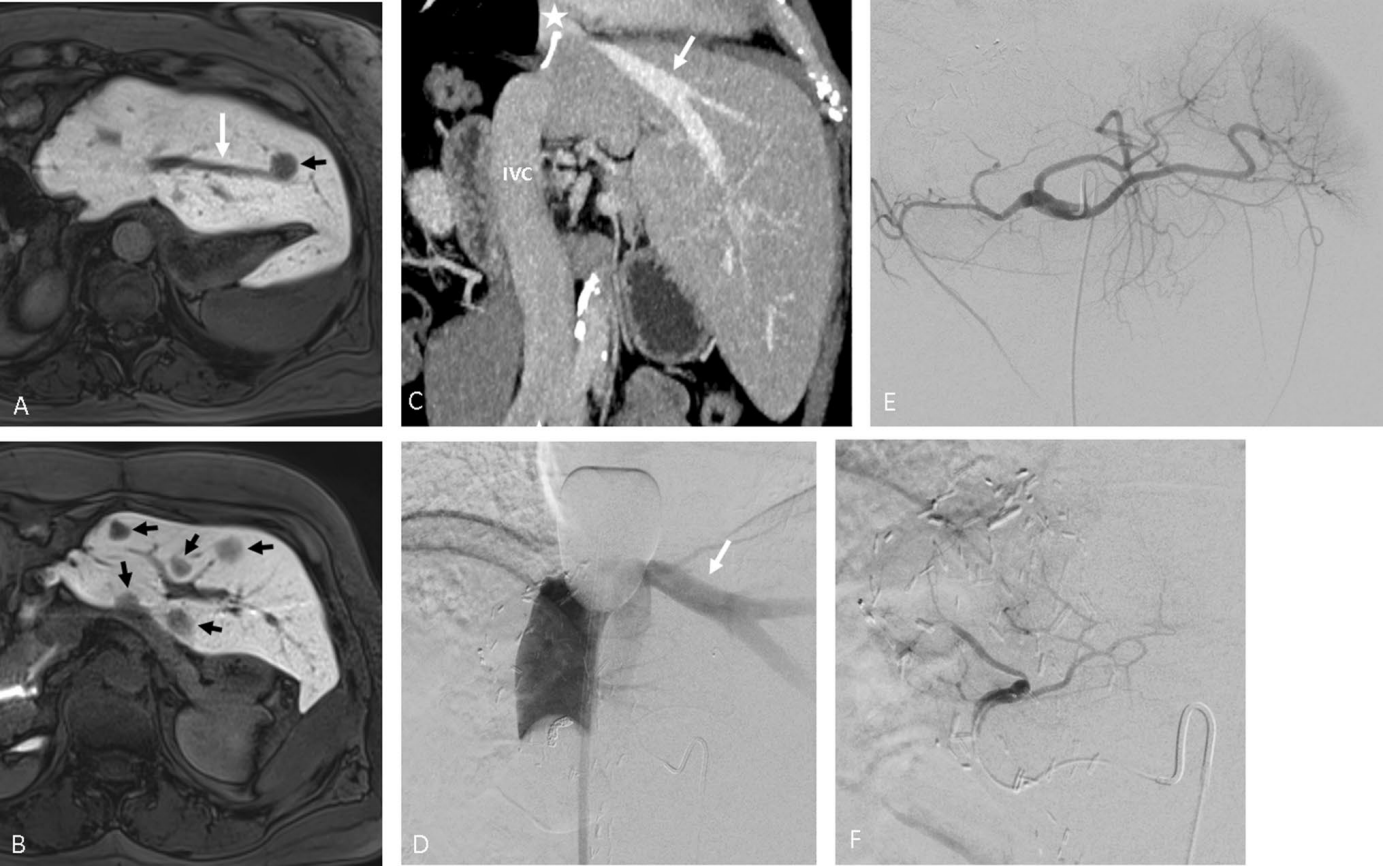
Gd-EOB DTPA-enhanced MRI of a patient with prior right hemihepatectomy. The multifocal hepatic metastasis of uveal melanoma in the left liver (black arrows) are clearly depicted in the delayed transversal T1 weighted phase (a, b). A coronal CT scan (c) gives an overview of the postoperative situs following right hemihepatectomy. The star (*) marks the confluens of the liver veins (white arrow) close to the resection margin. d Displays the retrograde injection of contrast agent during CS-PHP to verify correct placement of the double balloon catheter. The cranial balloon of the double balloon catheter is placed in the cavoatrial junction in close proximity to the resection margin. The caudal balloon is placed below the confluens of the liver veins. No leakage is visible while the left liver vein is opacified (white arrow). e Presents an overview angiography of the celiac trunk with a small left hepatic artery. The catheter used for administration of melphalan is advanced to the left hepatic artery (f). IVC = inferior vena cava
